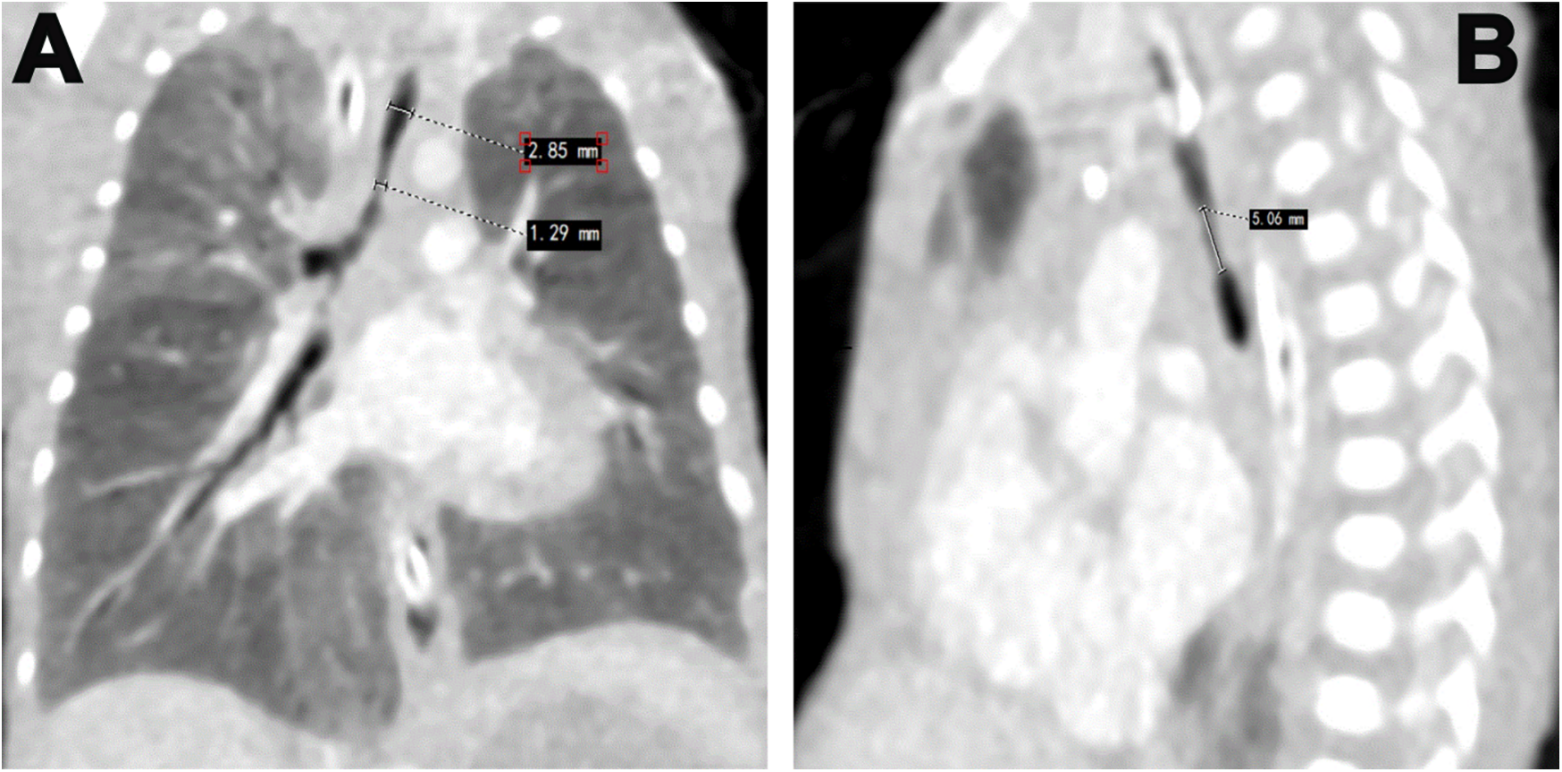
Enhance CT images showing: (A) the upper width of the trachea is 2.9 mm whereas the stenosed part has a width of 1.9 mm. (B) showing a length of stenosed part 5.06 mm.
